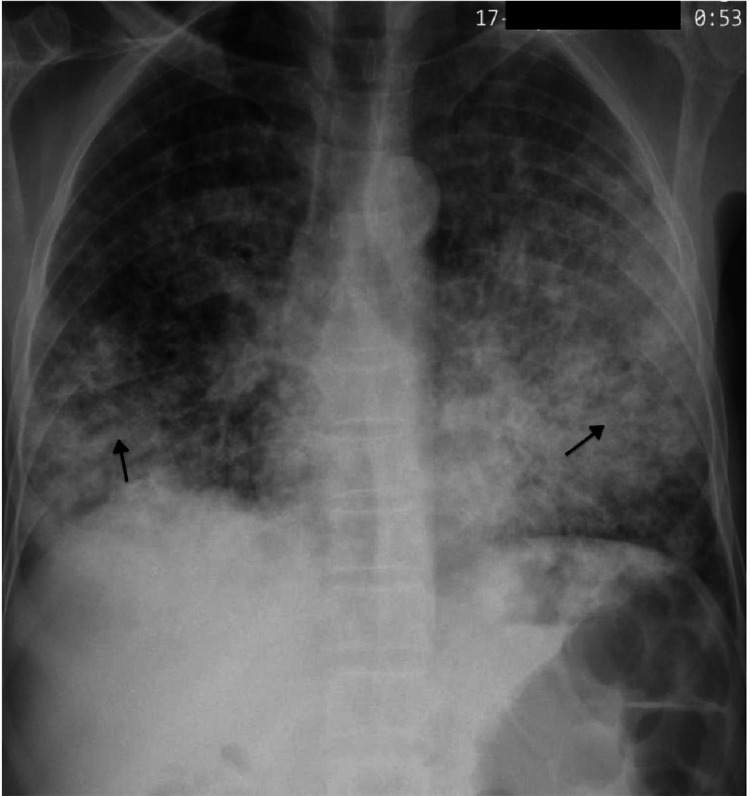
X-ray chest showing bilateral diffuse heterogeneous opacities (black arrows)
